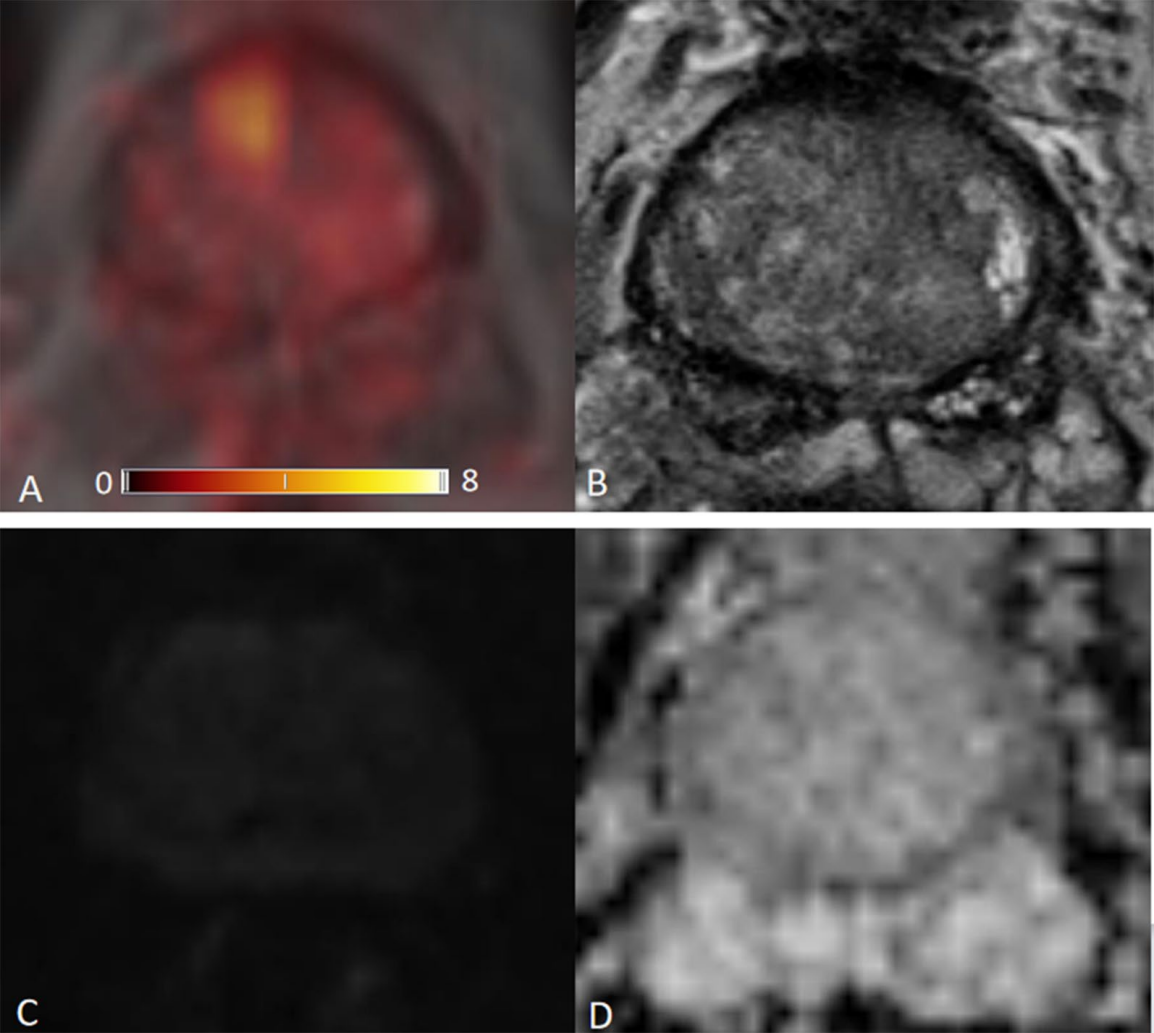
[^68^Ga]Ga-PSMA PET/MRI images obtained in a 63-year-old man, PSA 0.50 ng/mL. a [^68^Ga]Ga-PSMA PET/MRI; b T2WI; c DWI, *b* value 1000 s/mm^2^; d ADC. This example showed that normal prostate tissue and MRI-negative lesions show varying levels of PSMA uptake. The highest uptake in this figure is SUVmax 10.9, right anterior TZ, background SUVmax 1.1, LBR 9.9
